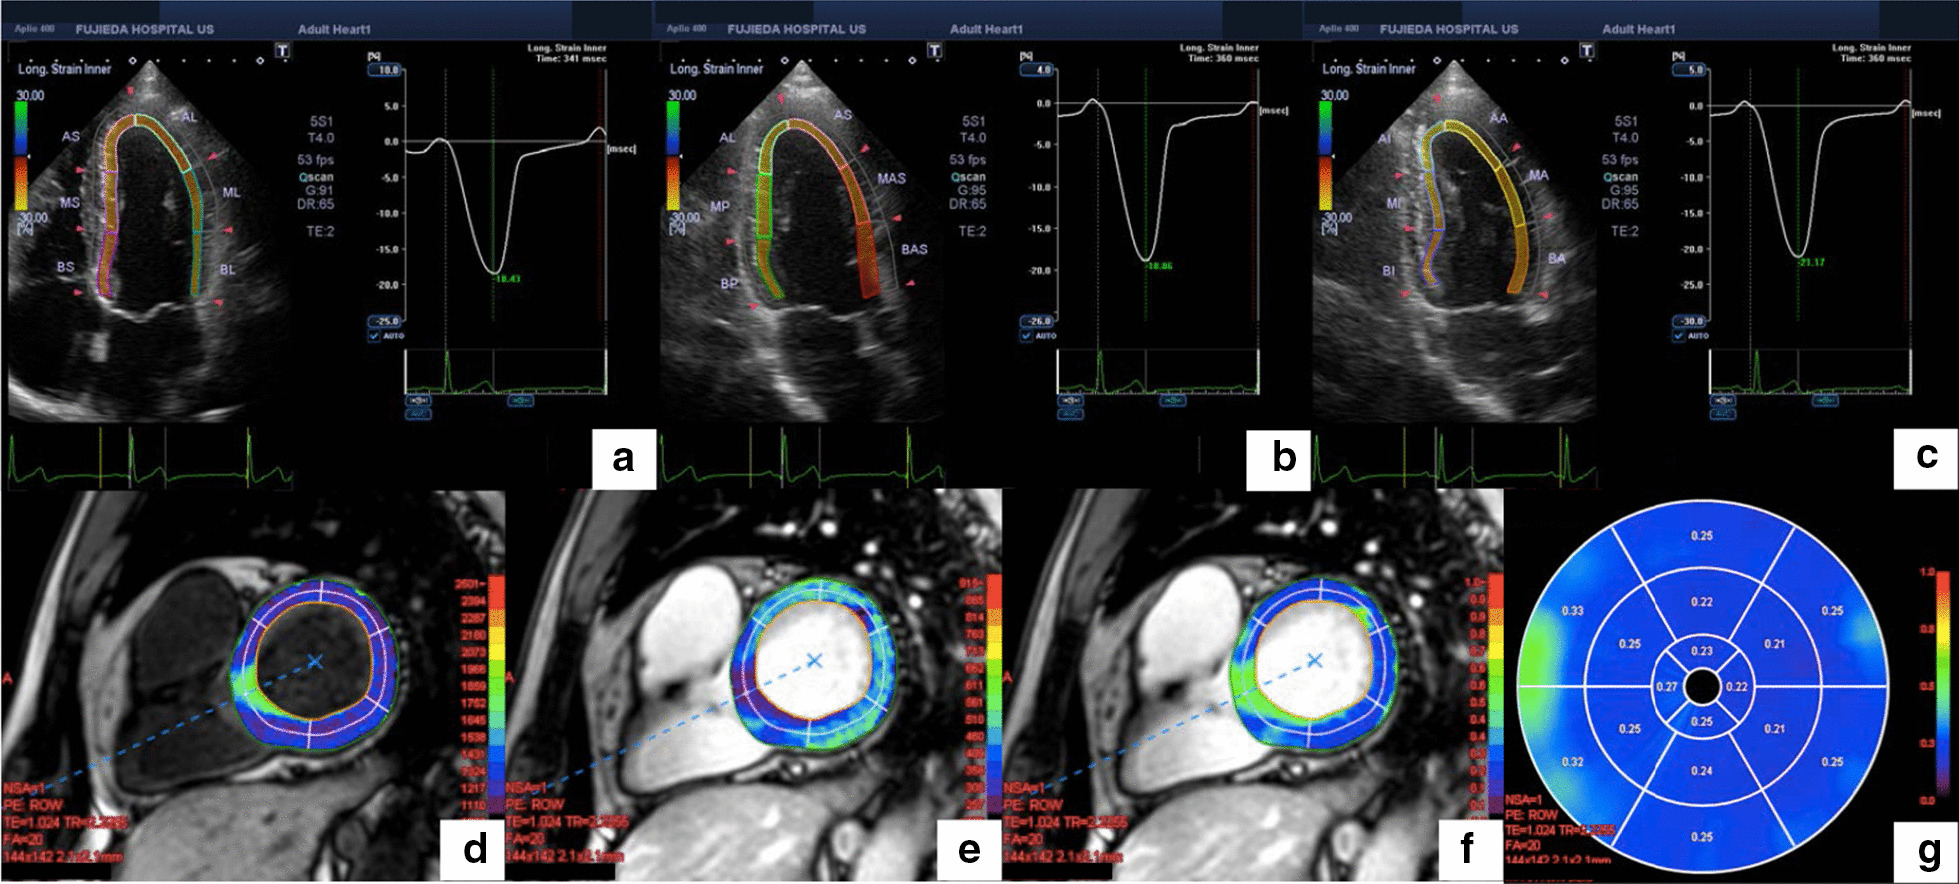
Example of assessment of left ventricular global longitudinal strain (LVGLS) and extracellular volume fraction (ECV). Apical four- (a), three- (b), and two-chamber (c) views of two-dimensional speckle-tracking echocardiography imaging are shown. LVGLS was calculated by averaging the values from these results. Maps of the LV basal short-axis segment with a modified Look-Locker inversion recovery sequence, native T1 mapping (d), post-contrast T1 mapping (e), and calculated ECV mapping (f) are shown. Global ECV value was calculated by averaging the values of the American Heart Association 16-segment model (g)
